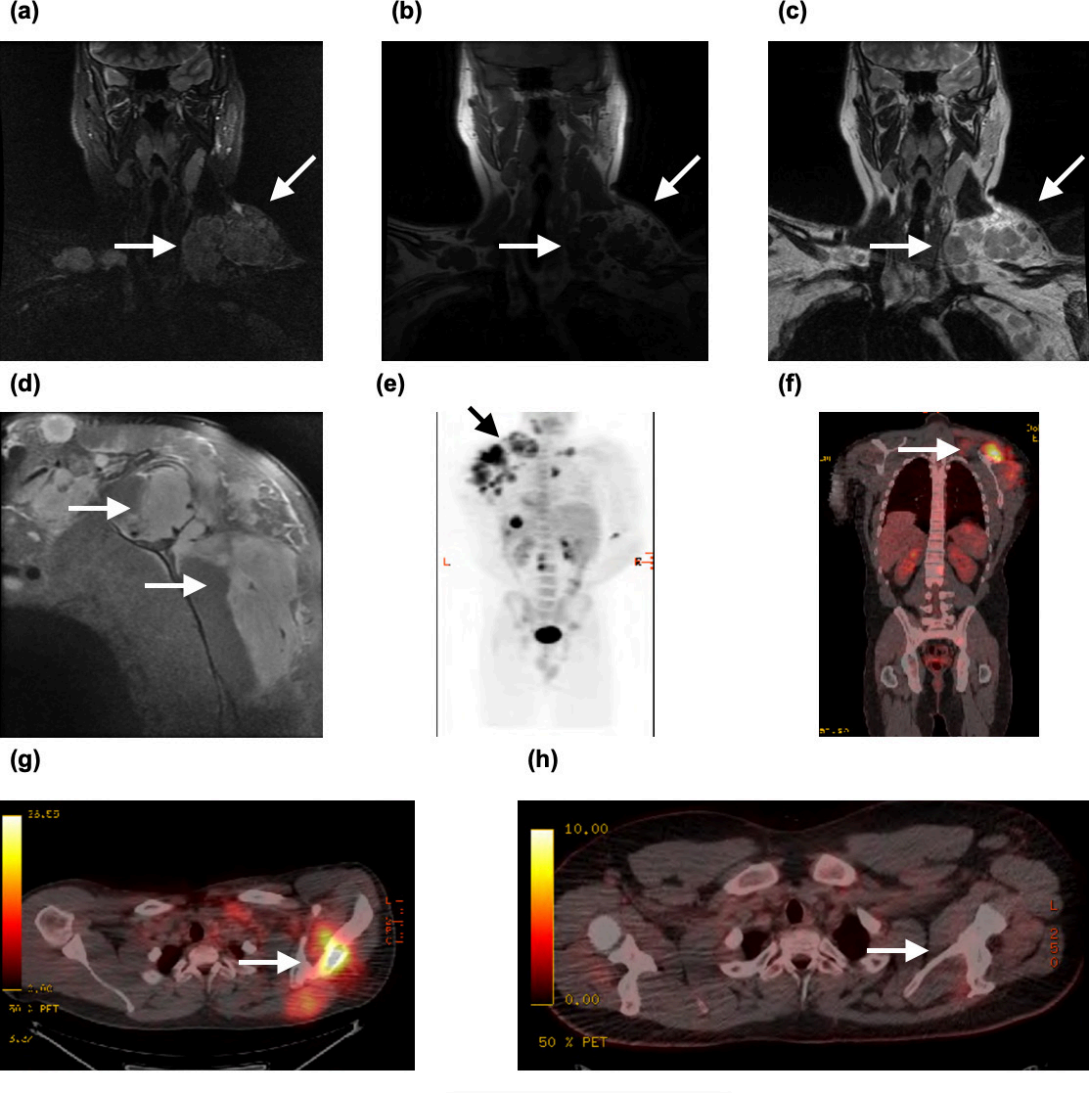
(a) Coronal STIR MR image of the left brachial plexus and shoulder showing a large volume conglomerate nodal mass with mixed density, (b) coronal *T*_1_ weighted MR image showing multiple enlarged lymph nodes which are isointense to skeletal muscle and hypointense to surrounding fat, (c) corresponding coronal *T*_2_ weighted MR image of the lymph nodes demonstrating intermediate intensity. (d) Sagittal fat-supressed MR image of the left shoulder showing enlarged hyperintense supraspinatus and infraspinatus muscles, (e, f, g) PET-CT image with corresponding FDG-avid lesions in the left shoulder/axilla and (h) end-of-treatment PET demonstrating complete resolution of disease. FDG, fludeoxyglucose; PET, positron emission tomography; STIR, short-tau inversion recovery.
